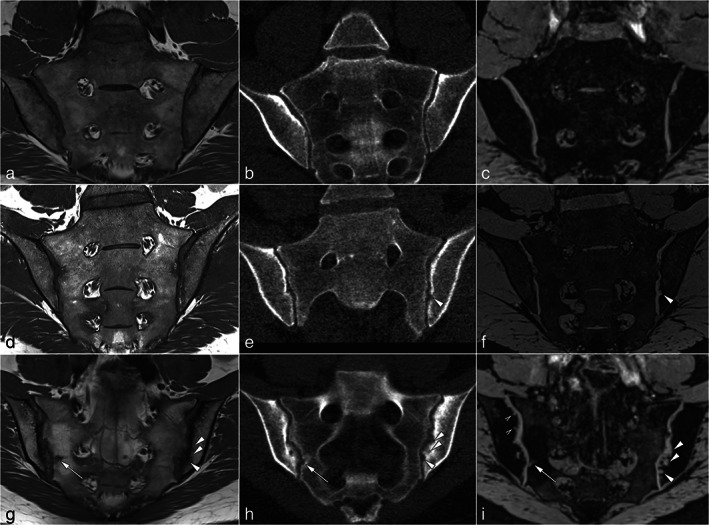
Imaging examples. (a, d, and g) Oblique coronal MR‐T1 sequence; (b, e, and h) low‐dose CT images in oblique coronal reconstruction; (c, f, and i) oblique coronal MR‐VIBE sequence. Slice positions and orientation are identical for T1 and VIBE. Low‐dose CT was reconstructed to match orientation and position. (a–c) normal findings in the sacroiliac joint without erosions. (d–f) Patient with axial spondyloarthritis with a single but prominent erosion of the left iliac surface that is shown by low‐dose CT and MR‐VIBE (arrowheads) but not by MR‐T1. (g–i) Patient with axial spondyloarthritis and multiple erosions. Some erosions (arrow) are depicted by all modalities. However, some larger erosions are hardly seen with MR‐T1 due to sclerosis, while they are more conspicuous using low‐dose CT and MR‐VIBE (arrowheads). The smallest erosions are only depicted with MR‐VIBE (open arrowheads). MR = magnetic resonance; CT = computed tomography; VIBE = volumetric interpolated breath‐hold examination. Reprinted with permission from reference 42.
